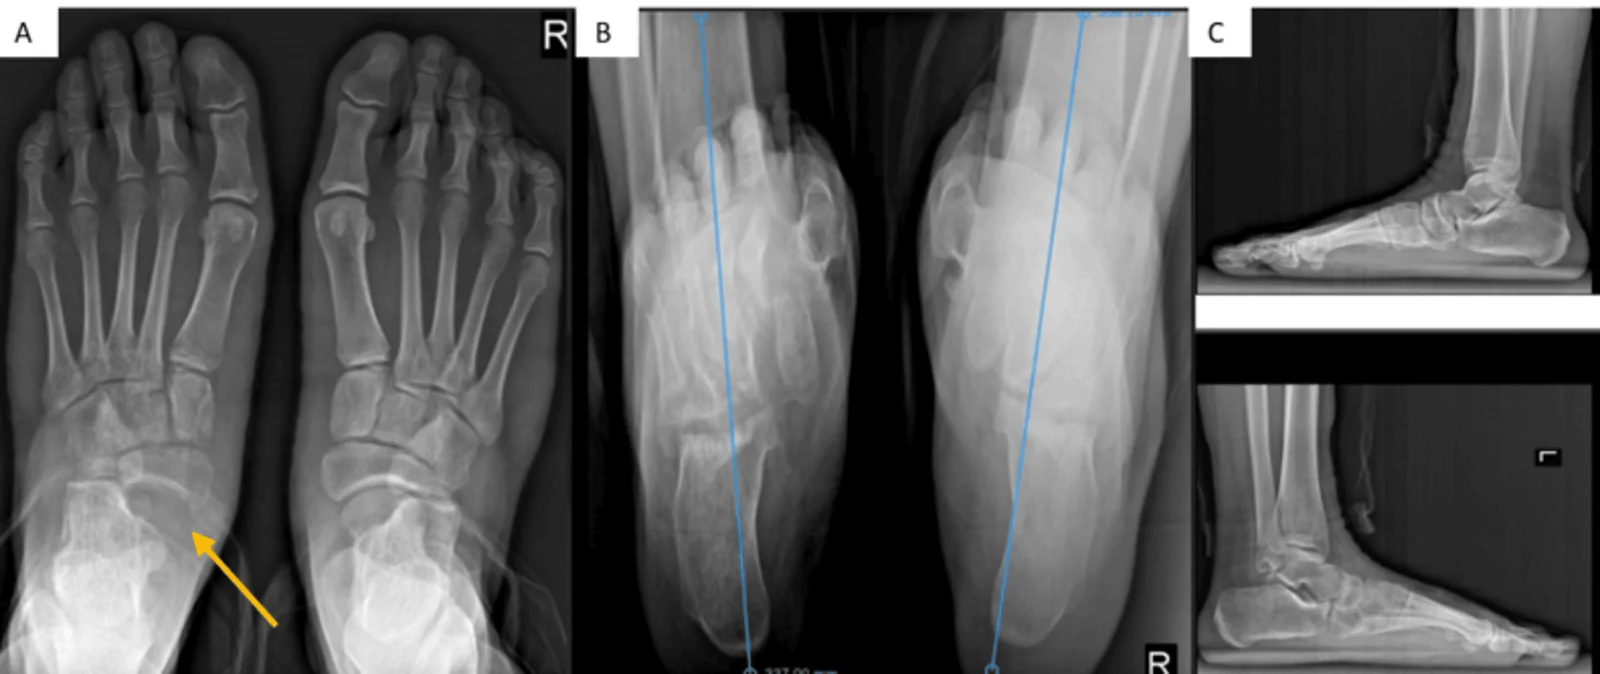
Preoperative radiographs taken three months after the injury upon referral to our clinic (A) Anteroposterior view of the foot (arrow showing left foot medial swivel dislocation), (B) Saltzman view, and (C) lateral view
